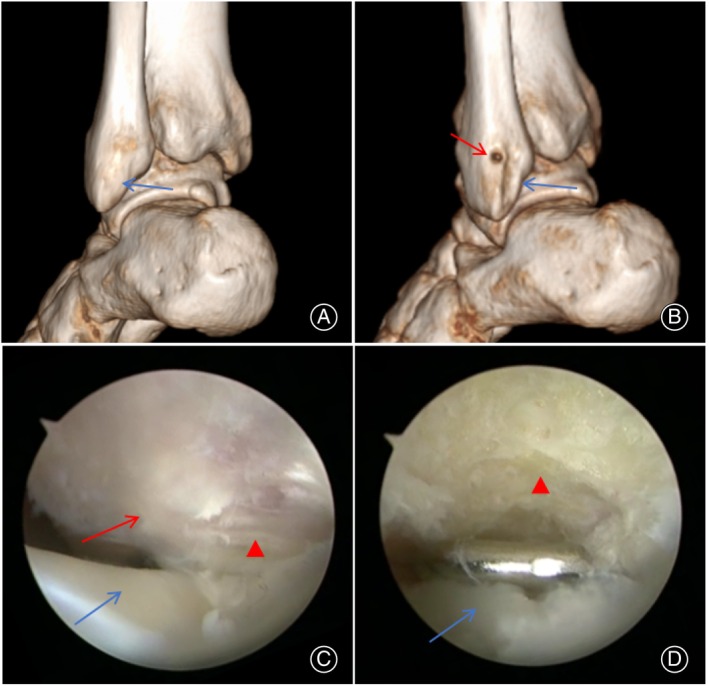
(A) Preoperative CT showed that the fibular groove was flat (blue arrow). (B) Postoperative deepening of the fibular groove (blue arrow) and the position of the anchor (red arrow). (C) Flat fibular groove (red arrowhead), SPR (red arrow), and peroneus longus tendon (blue arrow) under arthroscopy. (D) Deepening groove (red arrowhead) and peroneus brevis tendon removed the low‐lying muscle belly (blue arrow).
